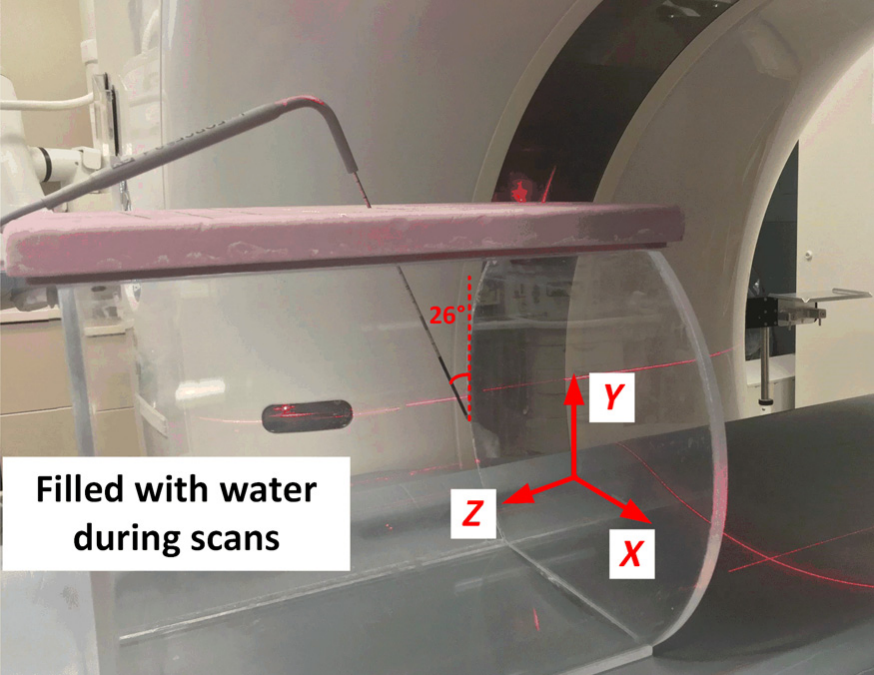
Experimental set-up of probe placement to collect CT images and create a digital probe model
